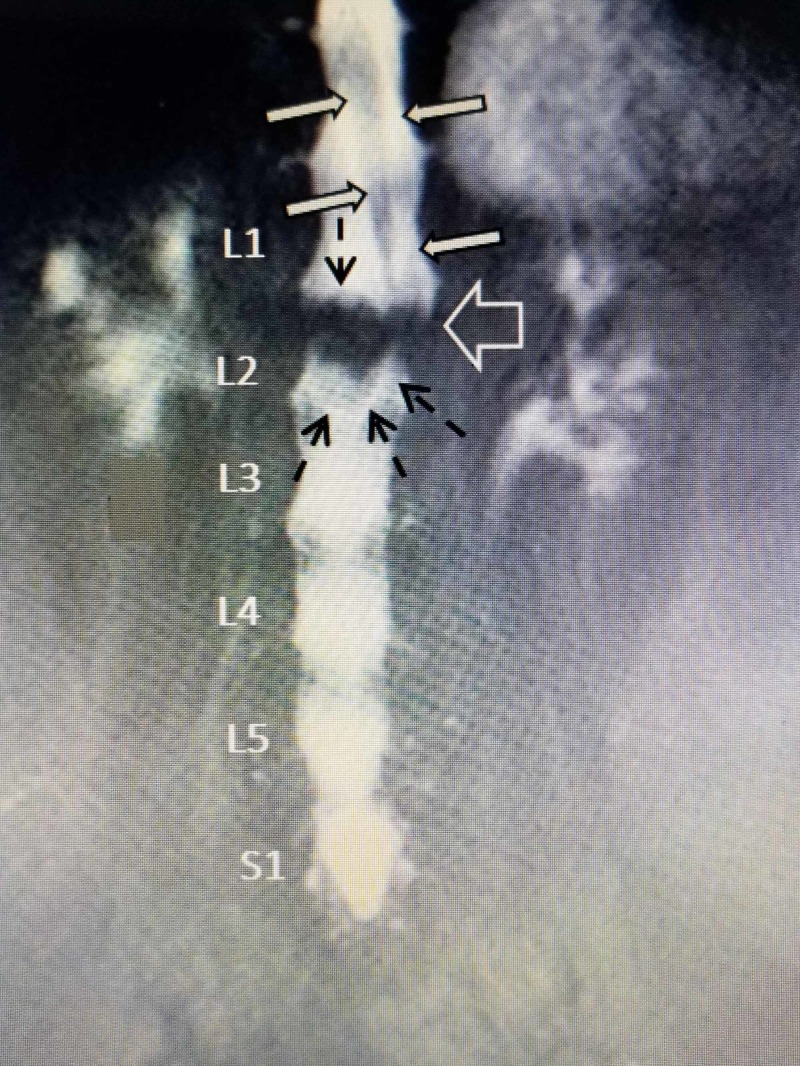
MRI myelogram showing severe localized obstruction of the CSF pattern at the L1-2 level with suggestion of an intraspinal mass The cerebrospinal fluid (CSF) obstruction is clearly seen in this coronal magnetic resonance imaging (MRI) at L1-2 (white border arrow). The upper lumbar nerve roots and conus medullaris are diverted (solid white arrows with black borders) away from a mass (solid black arrows). The outline of an oblong mass on the opposite side of the roots is noted (dashed black arrows).
